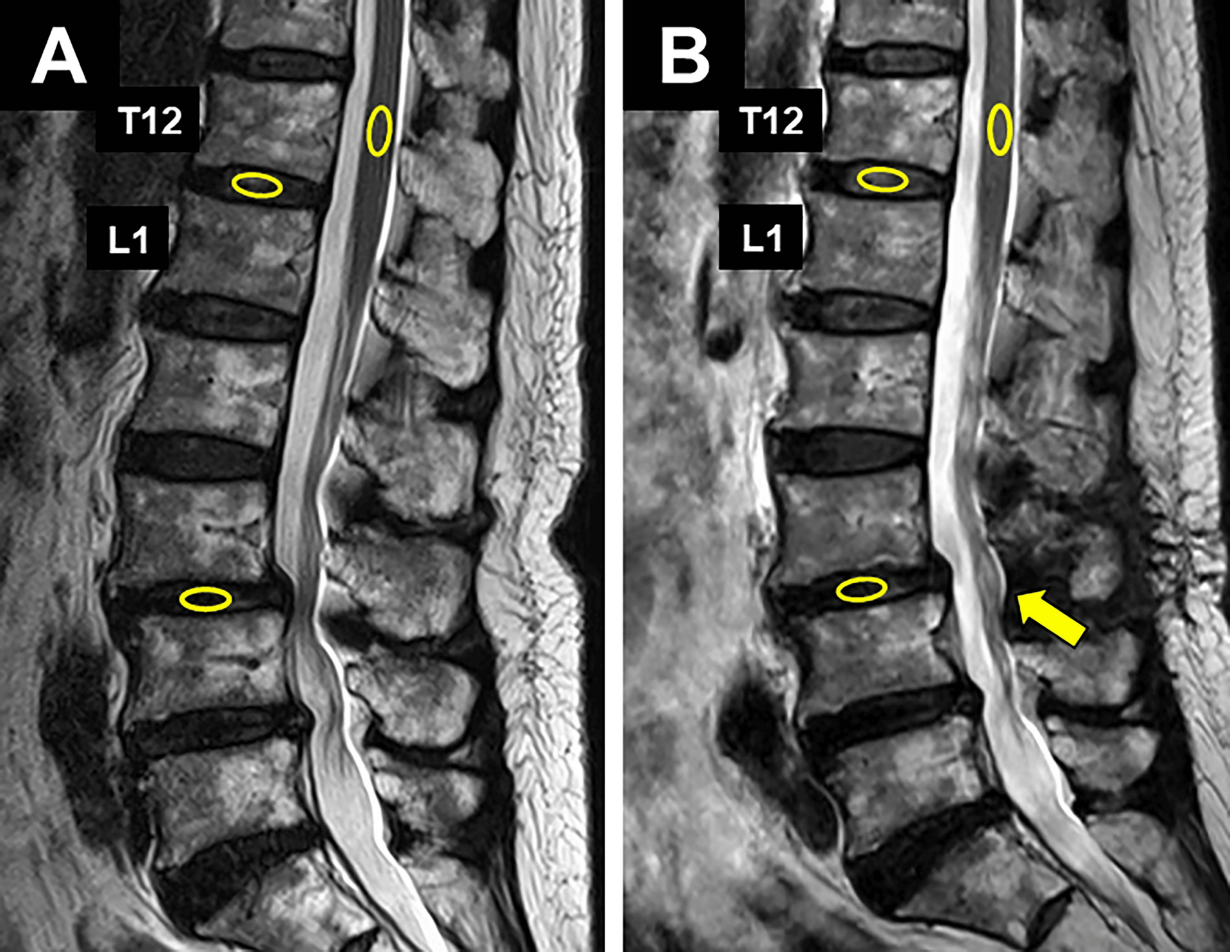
Area where the T2 signal intensities were measured Yellow ellipses on the midsagittal T2-weighted images indicate regions of interest at the center of the disc (T12-L1, decompression level) and in the conus medullaris of the spinal cord at the T12 vertebral level. The yellow arrow indicates the site where posterior decompression was performed. Preoperative MRI (A), postoperative MRI (B)
